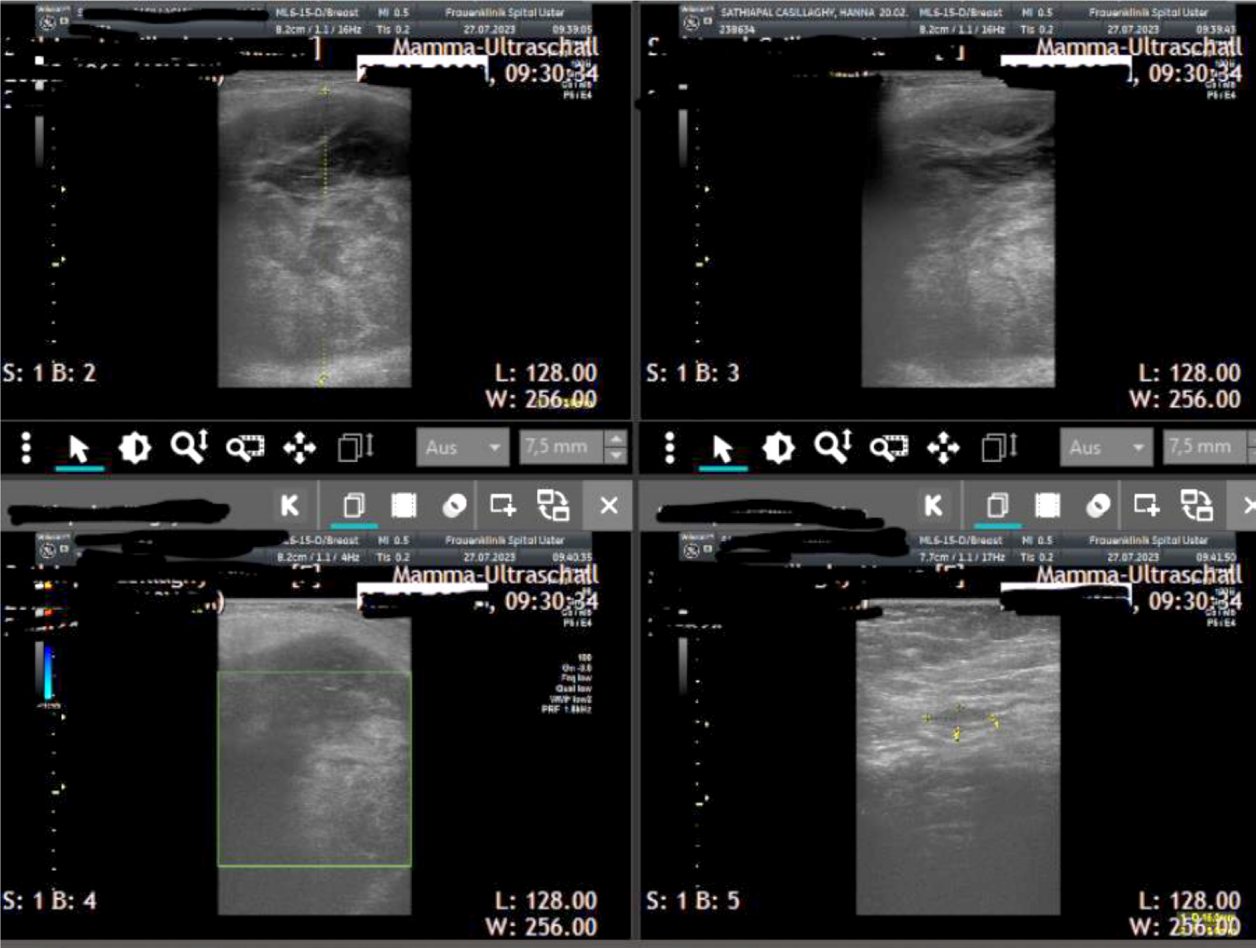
Ultrasonography results. Case 2
